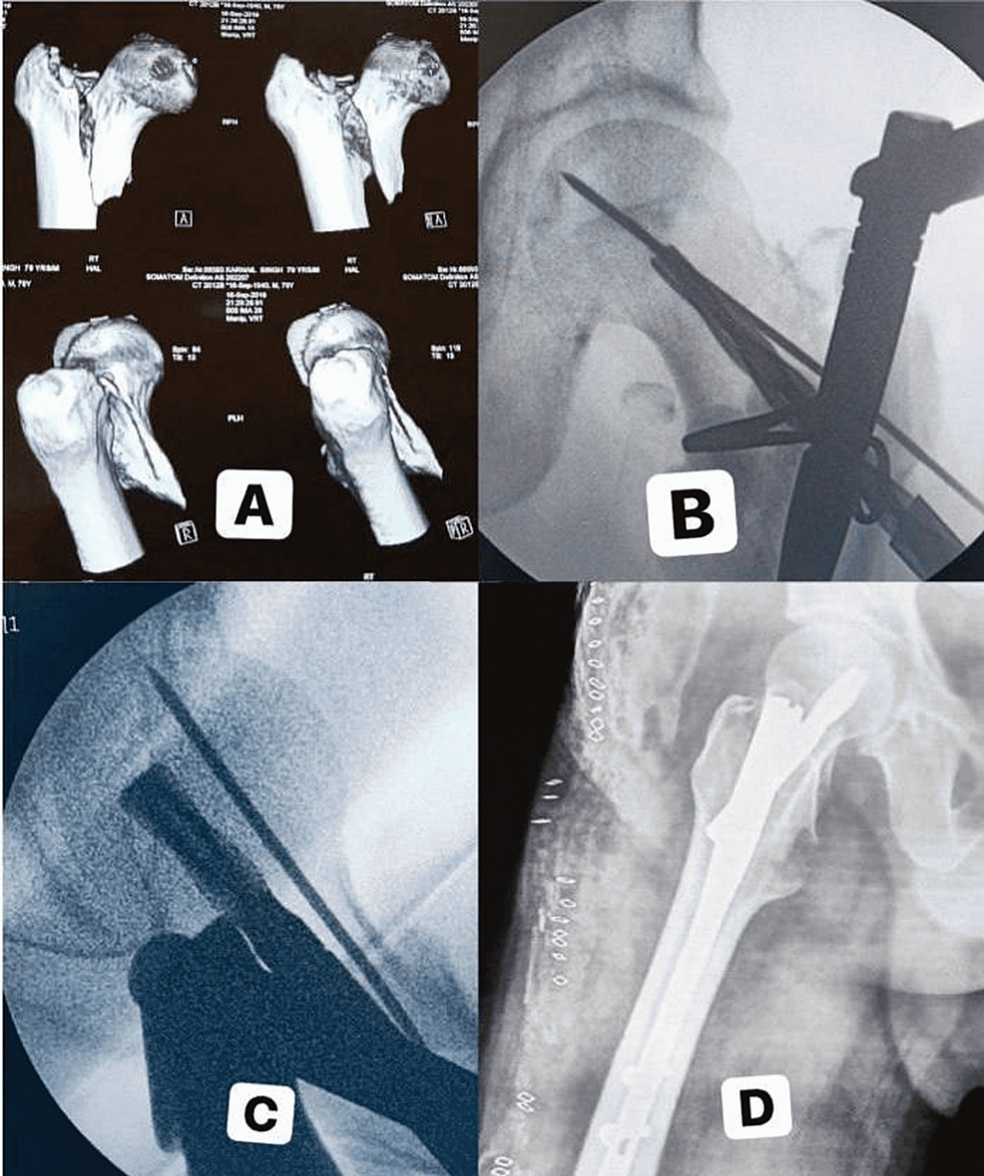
Case two - reduction techniques for unstable intertrochanteric fracture A: 3D CT images of fracture in a 62-year-old patient; B, C: Anteroposterior (AP) and lateral radiographic view of fracture reduction using Kirchner wires placed anteriorly; D: Lateral view showing a good postoperative reduction
